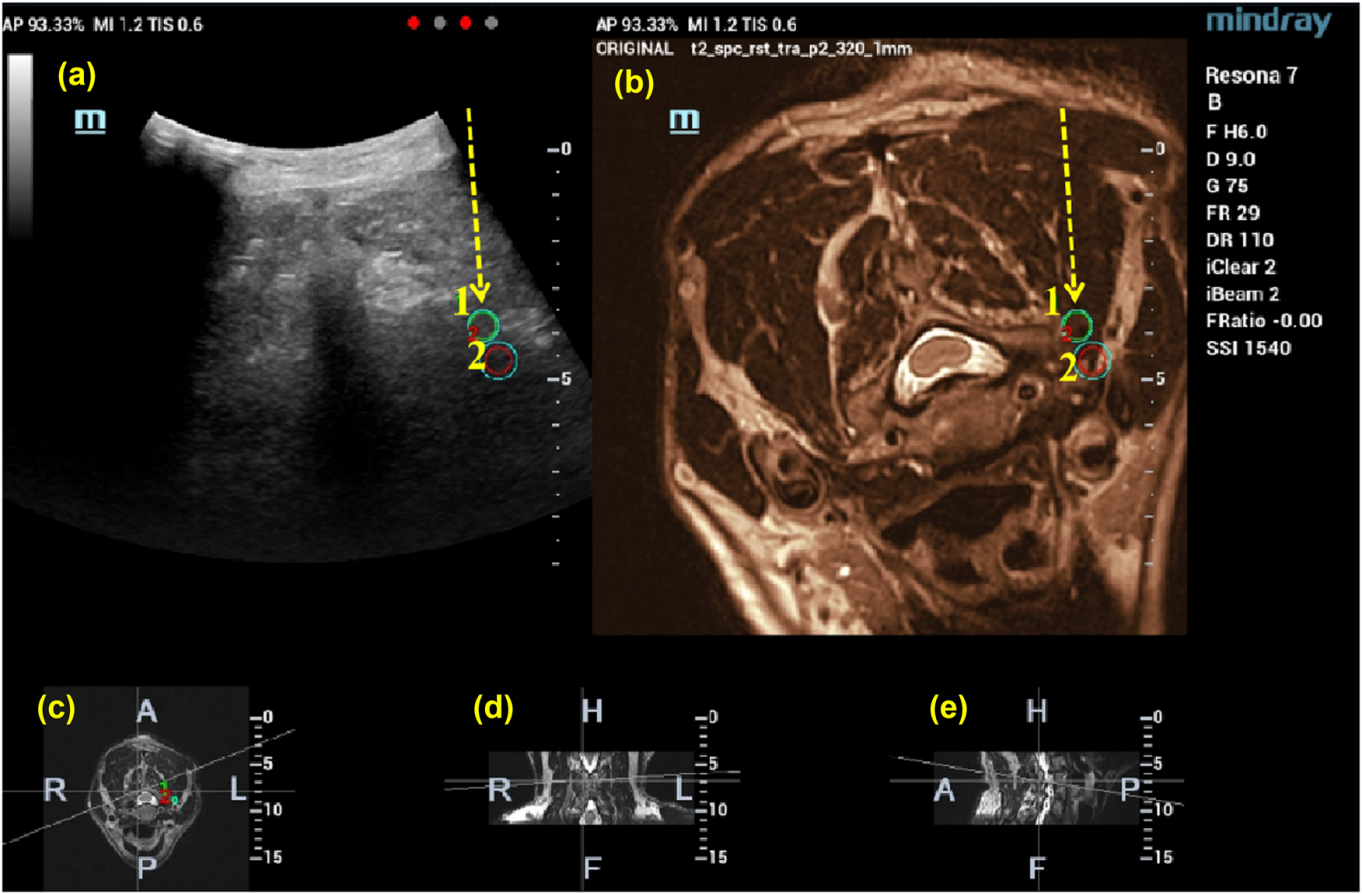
The therapeutic target is marked on the MRI image, and the location of the target is also displayed on the fused image at the same time (1, 2). Then, the puncture path is designed according to the MRI and US images (shown by the yellow arrow). (a) US-MRI fusion images, with green circles indicating the target puncture points, (b)MRI images, (c) cross section, (d) coronal section, and (e) sagittal section.
